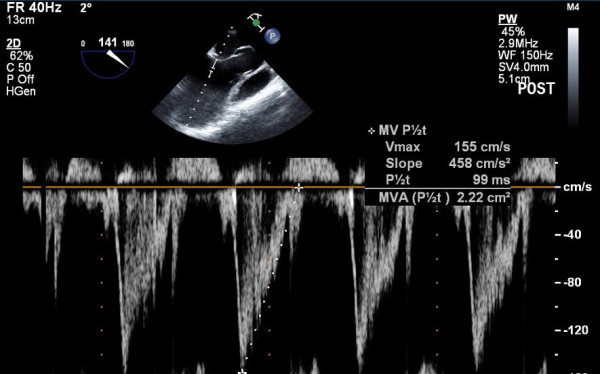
Determination of mitral valve area using pressure half-time with echocardiography.
